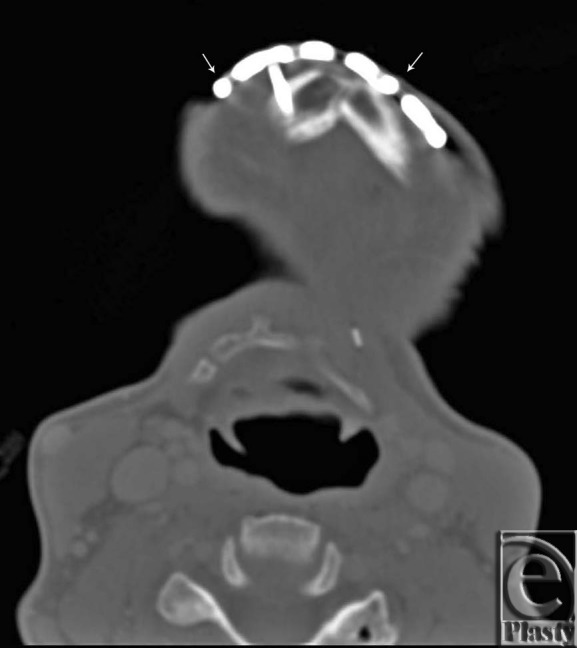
Preoperative computed tomographic scan. Arrows indicated where cuts were made with large surgical pin cutters to remove the exposed portion of the reconstruction plate.
